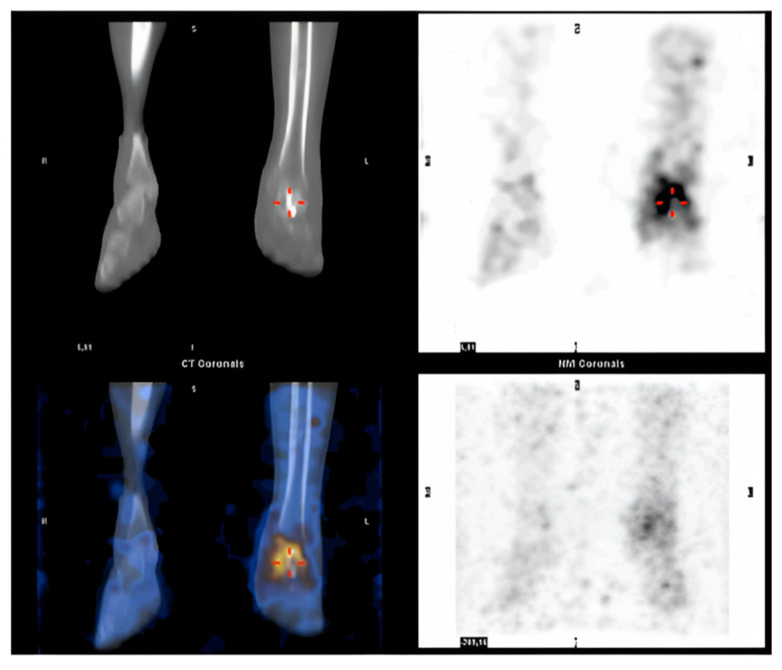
SPECT CT suggesting a deep tissue infection of the reimplanted talus. Red hyphens point out the site of infection.
